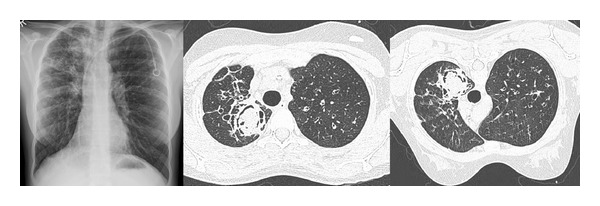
A pulmonary aspergilloma in a 24-year-old patient with cystic fibrosis. CT images show a fungus ball within the preexisting left upper lobe cavity, and the air-crescent sign is demonstrated in the nondependent part of the cavity on both CT imaging performed in the supine and prone position.
